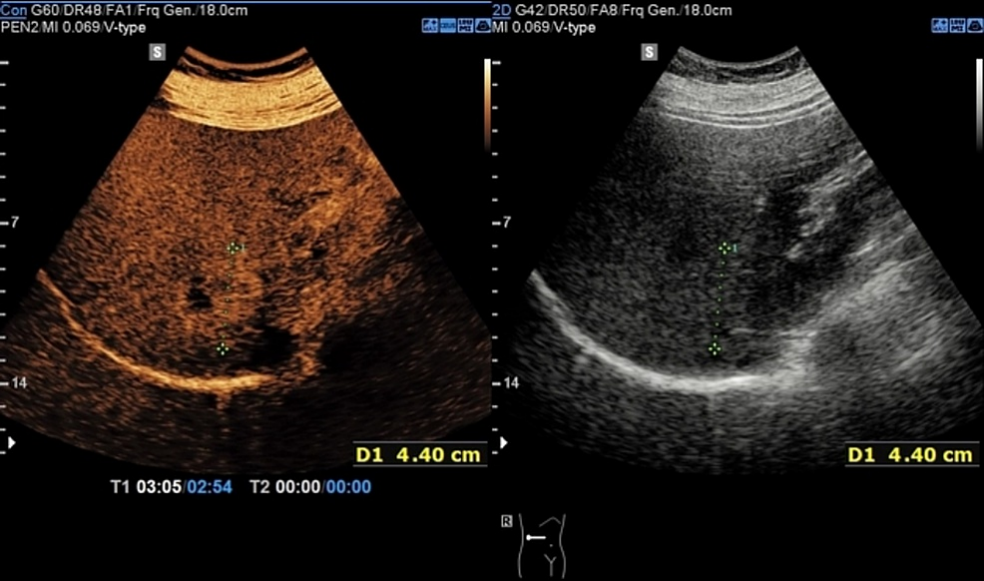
The liver lesion is barely seen on conventional B-mode US (on the right) compared to early arterial hyperenhancement (in the early post contrast phase) seen with CEUS using IV SonoVue (on the left). CEUS: contrast-enhanced ultrasound Image from Radiopaedia [18].
